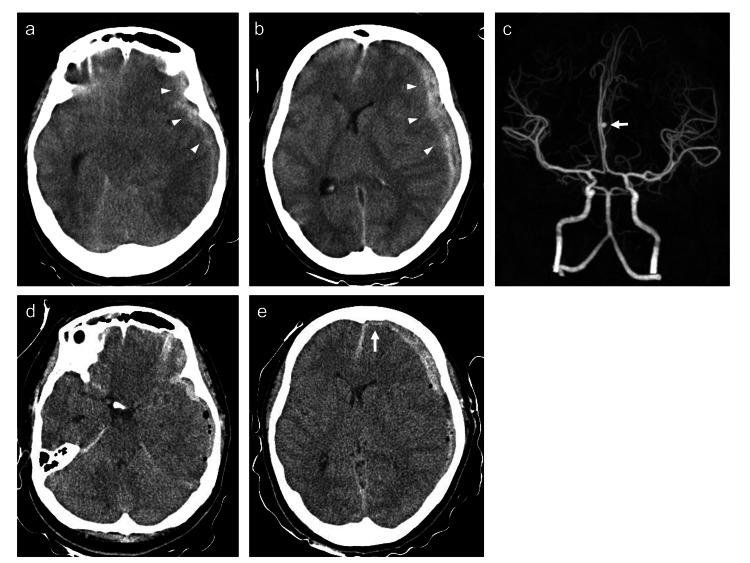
Imaging findings based on our patient with a pure acute subdural hematoma (ASDH) before the operation. (a, b) Axial computed tomography (CT) images obtained on admission showing a left convexity ASDH (arrowheads) with uncal herniation, with no evidence of subarachnoid hemorrhage (SAH). (c) CT angiography image showing a 4 mm right distal anterior cerebral artery (ACA) aneurysm (arrow). (d, e) CT just after hematoma evacuation using burr hole showing improvement of uncal herniation without SAH and connectivity of ASDH between interhemispheric fissure and left convexity space (arrow).
